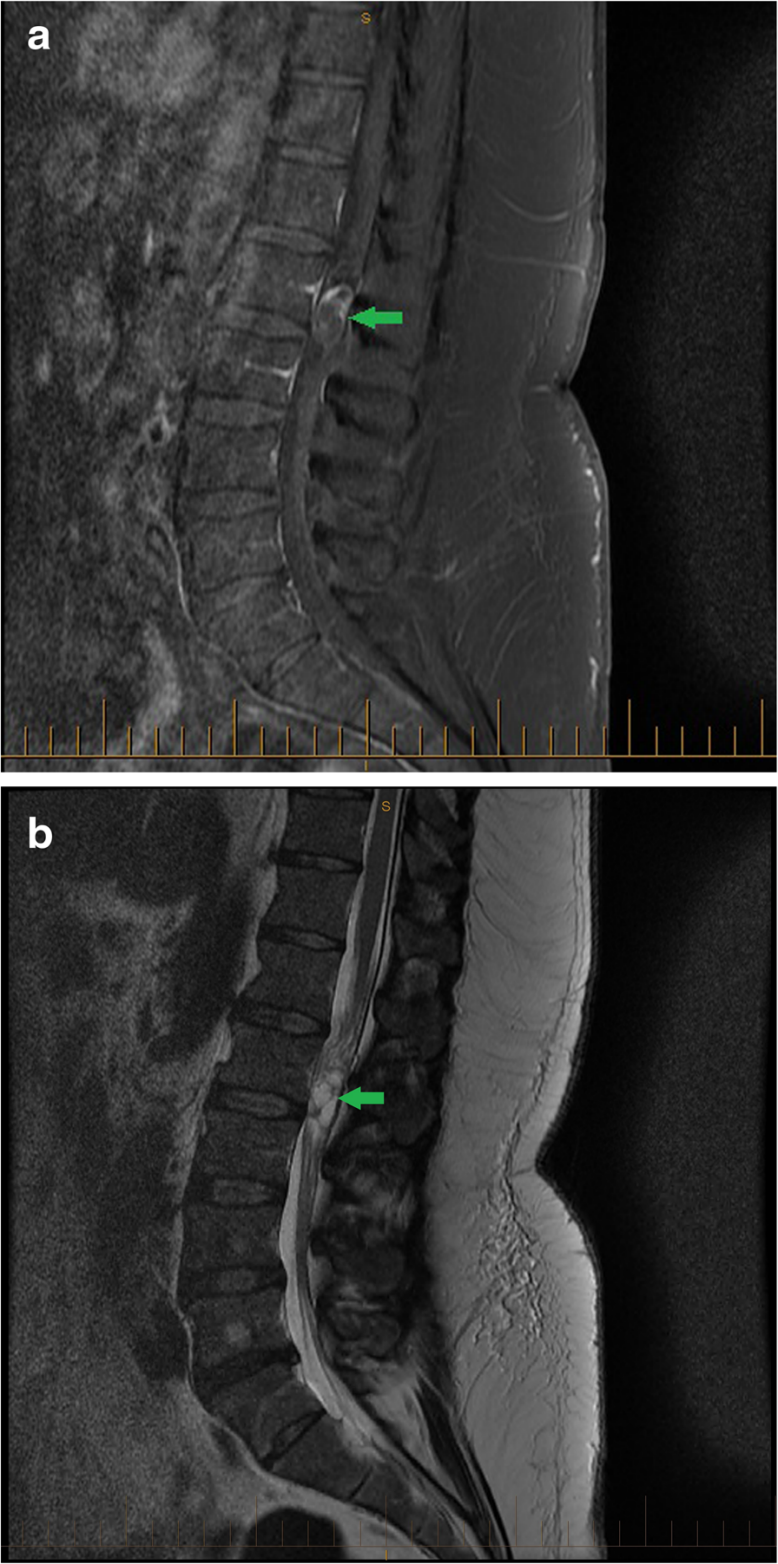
Sagittal fat suppressed T1-weighted (a) and T2-weighted (b) magnetic resonance images of the lumbosacral spine. A heterogeneous, predominantly cystic, intramedullary space-occupying lesion measuring 2.5 × 1.2 cm is present within the filum terminale at L2/3 level (green arrow). Additional findings include a transitional lumbosacral vertebra (lumbarisation of S1), dehydration of the L5/S1 intervertebral disc with a posterior disc bulge, and a hemangioma in the vertebral body of L5. The remainder of the spinal cord and conus medullaris, lower thoracic and lumbosacral vertebrae, intervertebral disc spaces, and paraspinal soft tissues appear normal
